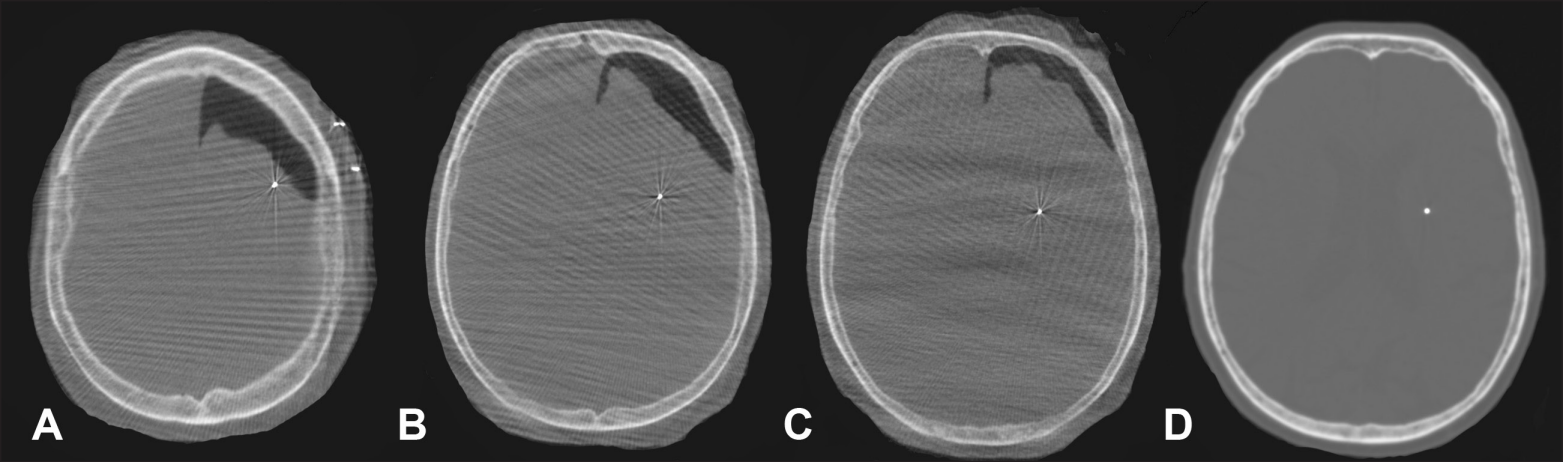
A, B, C: Different cuts of CT scan done immediately after lead implantation. There is significant left frontal pneumocephalus leading to brain shift in the posterior and medial direction. D: Resolved pneumocephalus at a four-weeks interval.
